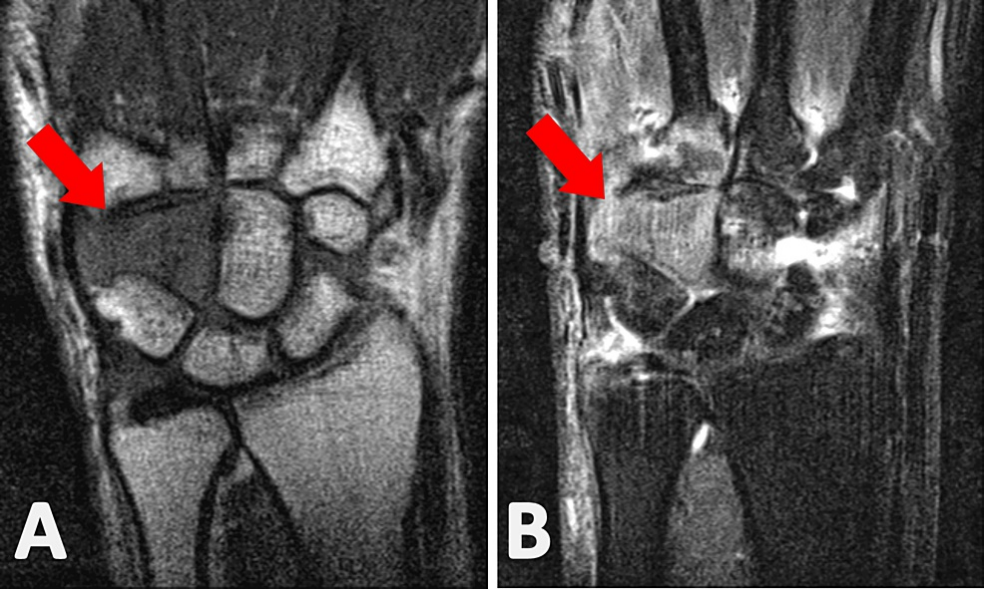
T1 (image A) and T2 (image B) weighted coronal MRI scans of the left wrist, eight weeks from symptom onset. The abnormal signal around the hamate bone area, shown by the arrows, was reported as an inflammatory mass.
